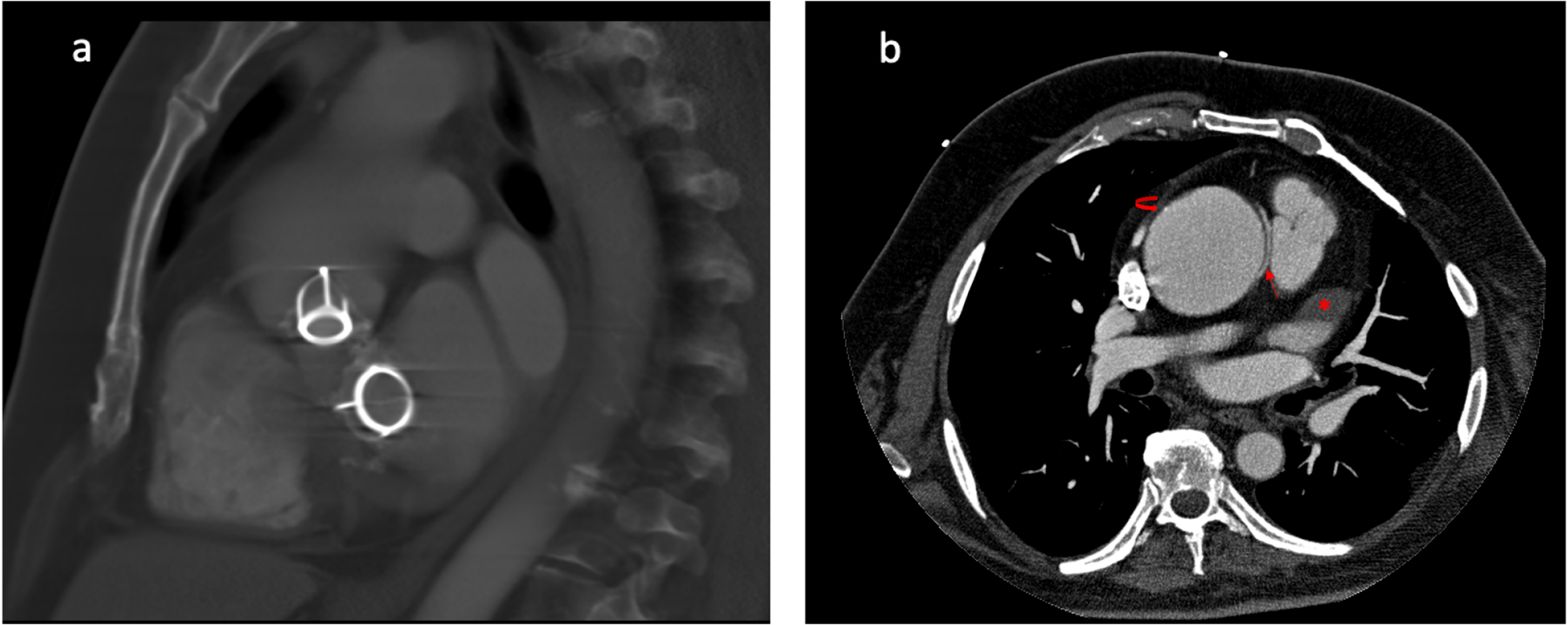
Cardiac Computed Tomography. Sagittal reconstruction showing both mitral and aortic silastic Starr-Edwards valves (1a); axial view showing ascending aortic dilation (curved arrow), the anomalous origin of the right coronary artery (straight arrow) coursing between the aortic root and the main pulmonary artery and a thrombus in the left atrial appendage (asterisk) (1b)
